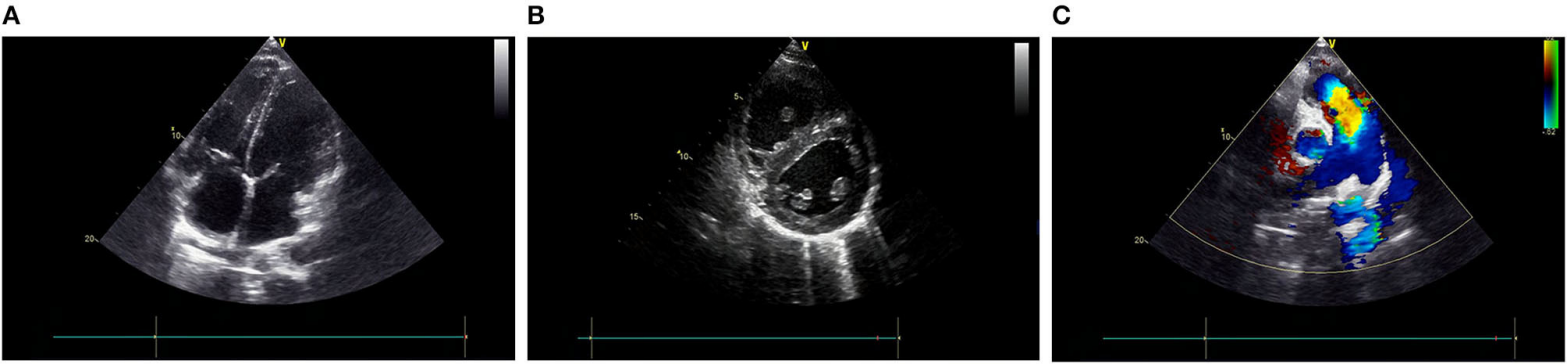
Echocardiography shows both RA and RV are significantly dilated, and the RV wall thickness is increased (A,B). An enlargement of the PA and (C) its branches. PA, pulmonary artery; RPA, right pulmonary artery; LPA, left pulmonary artery; AO, aorta; RA, right atrium; RV, right ventricle; LA, left atrium; LV, left ventricle.
